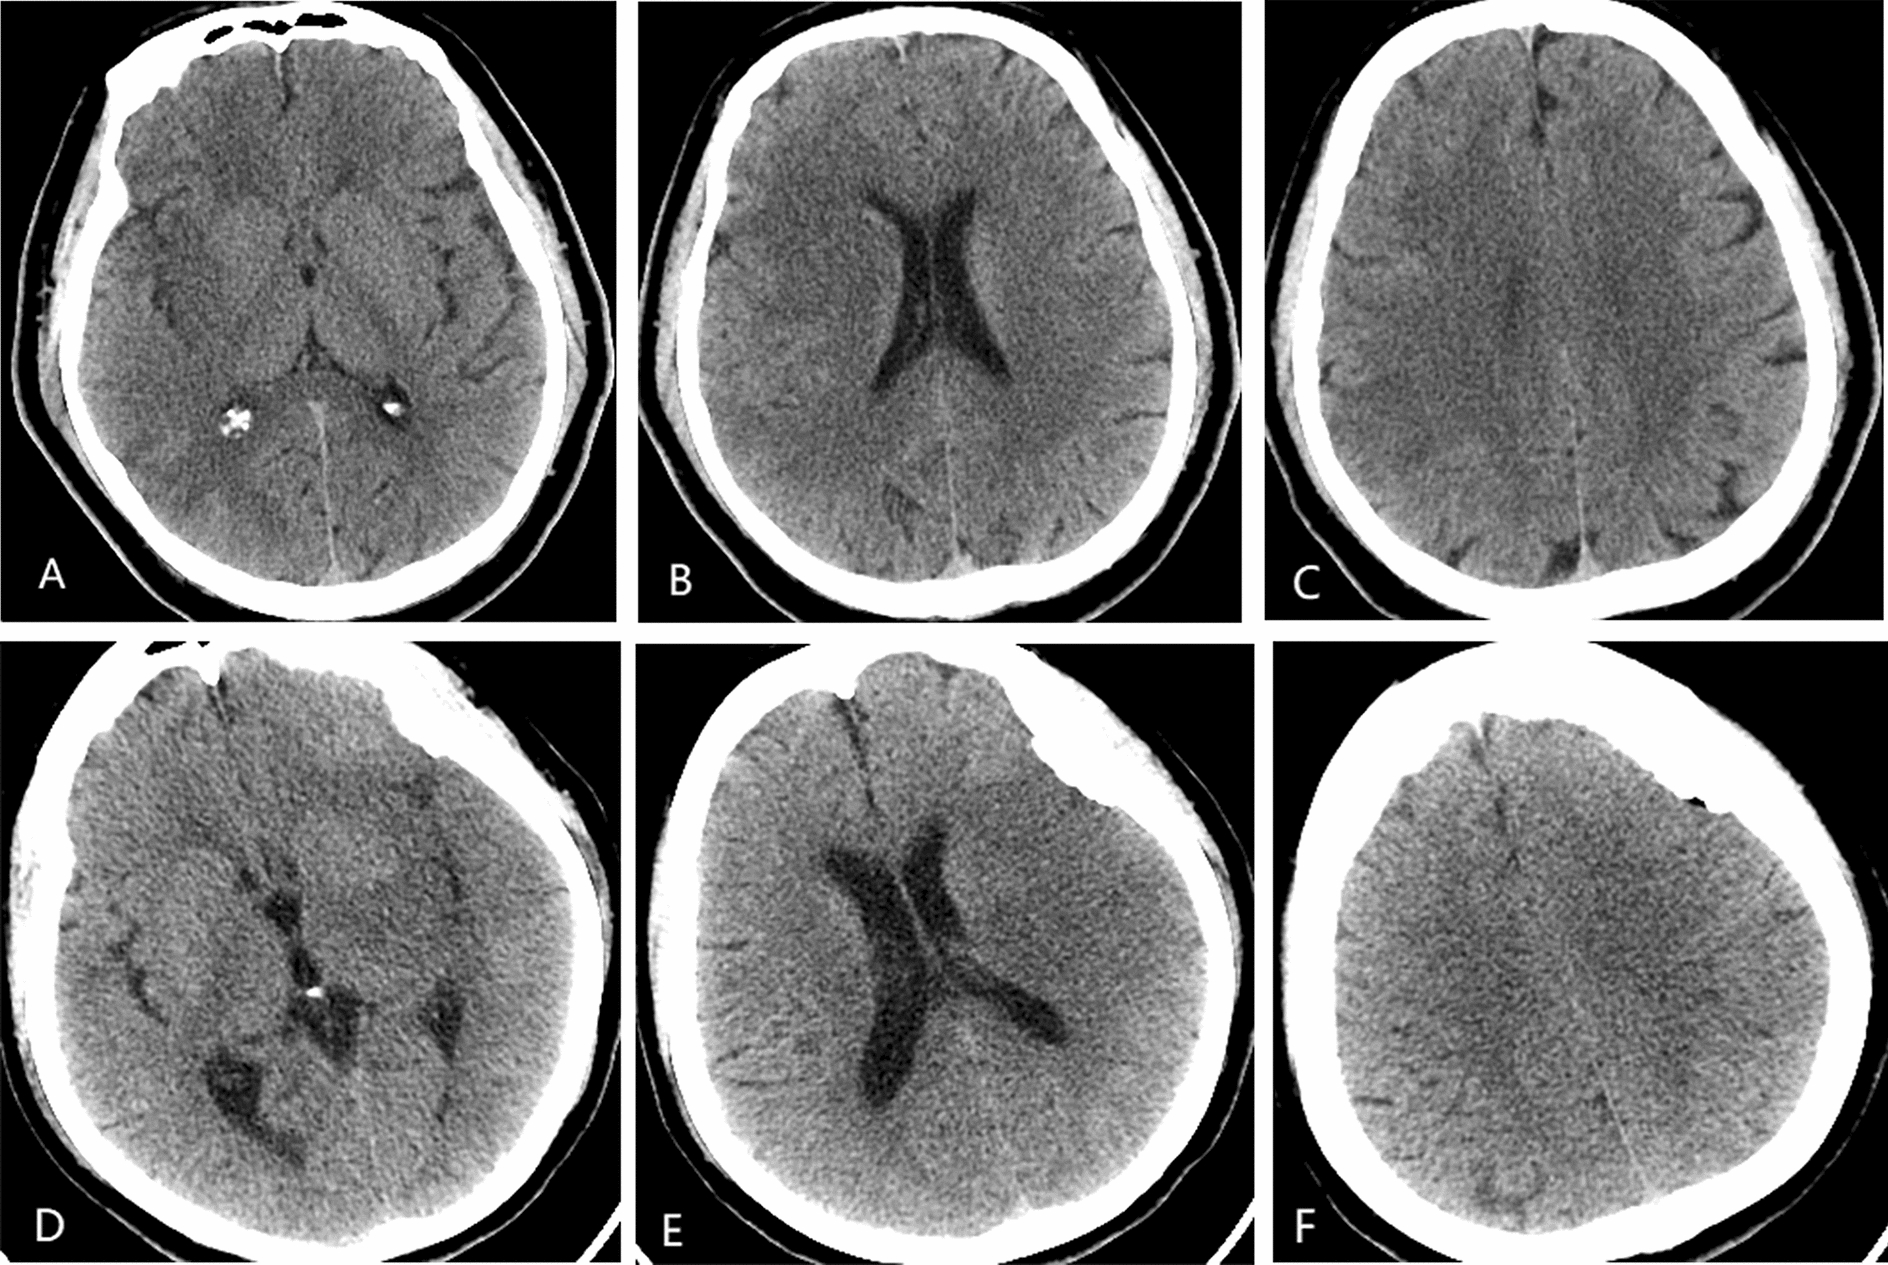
Representative images of patients in ASPECTS tiers: A–C 56-year-old man with baseline ASPECTS of 6 (M1, M2, L, M6); baseline NIHSS score, 11; HbA1c, 9.22; mTICI score, 2b; and mRS score, 2. D–F 77-year-old woman with baseline ASPECTS of 4(M2, I, L, IC, M5, M6); baseline NIHSS score, 20; HbA1c, 5.38; mTICI score, 2b; and mRS score, 3. Middle cerebral artery (MCA), insula (I), lenticular nucleus (L), caudate nucleus (C), posterior limb of internal capsule (IC)
